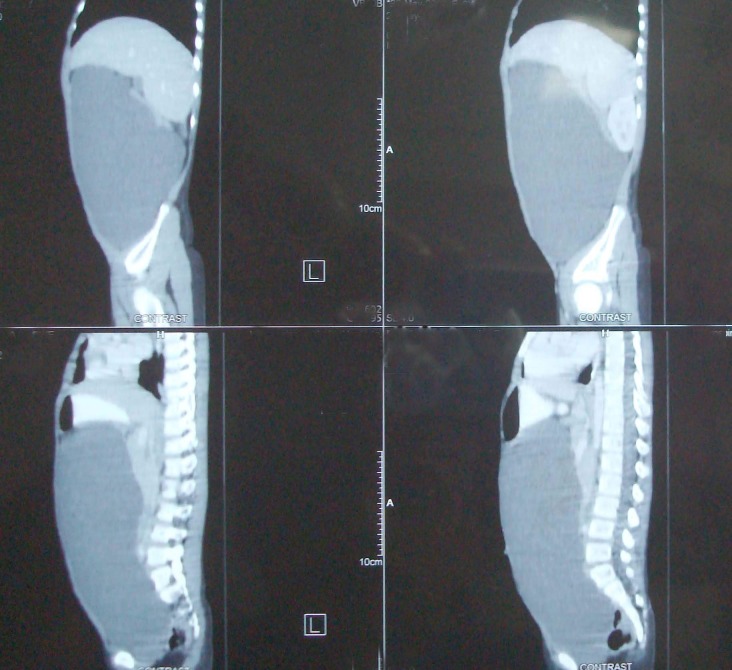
Figure 1: CT scan, saggital section showing huge unilocular cystic abdominal mass.
